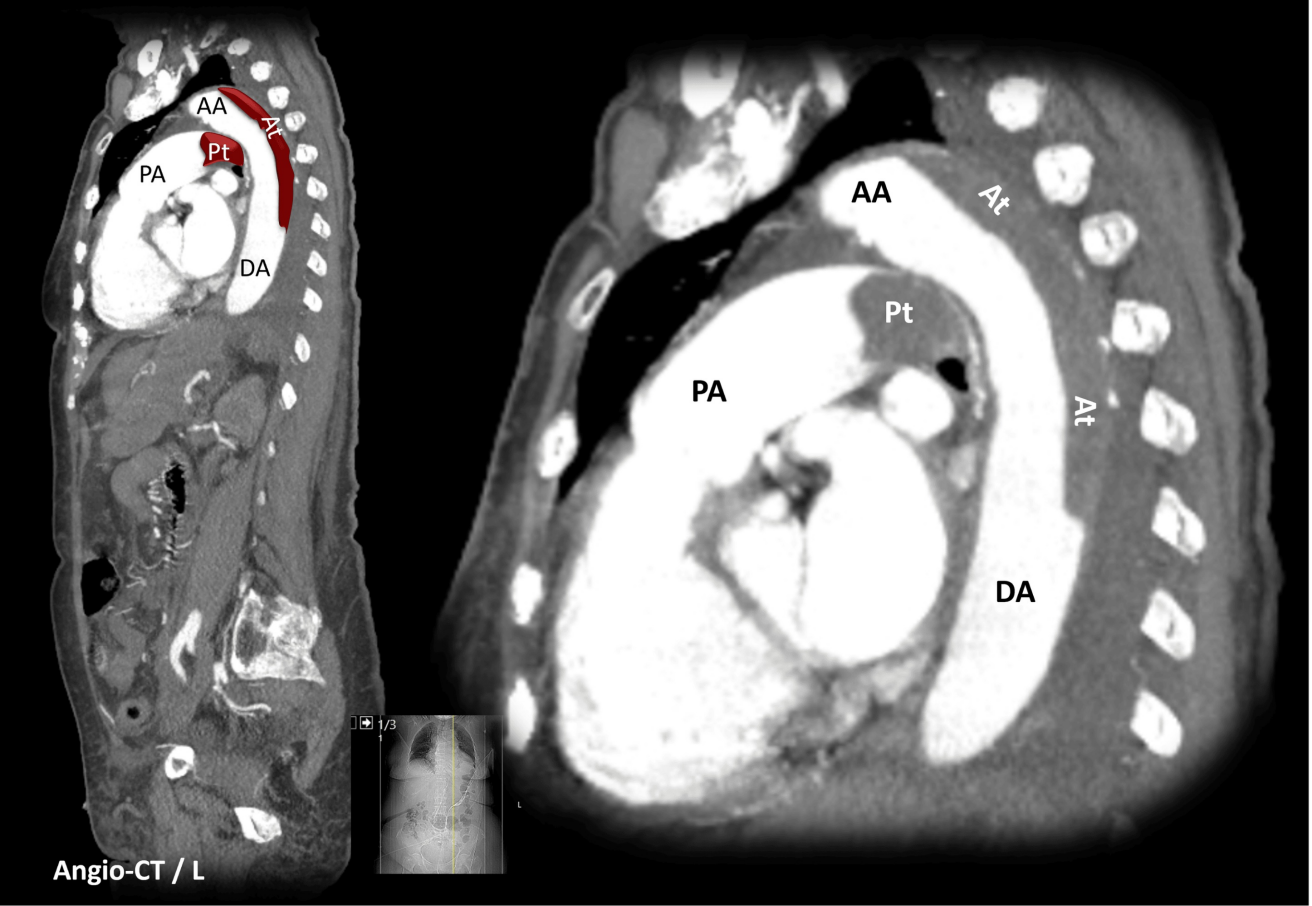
Chest angio computed tomography (longitudinal reformatting) AA - Ascending Aorta; At - Aortic Thrombosis; DA - Descending Aorta; PA - Pulmonary Artery; Pt - Pulmonary Thrombosis
